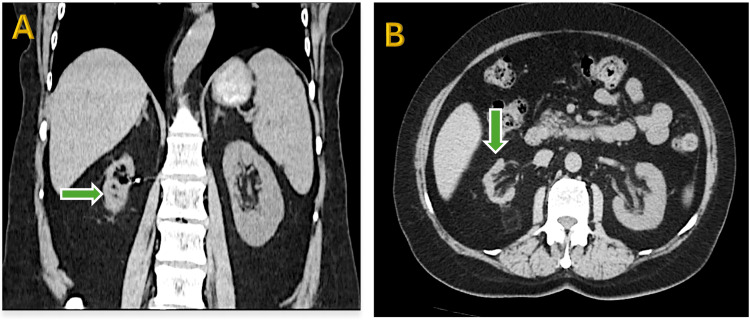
Follow-up CT of the kidneys showing right renal atrophy. (A) Coronal section: Green arrow indicates the atrophic right kidney. (B) Axial section: Green arrow highlights the atrophic right kidney.
